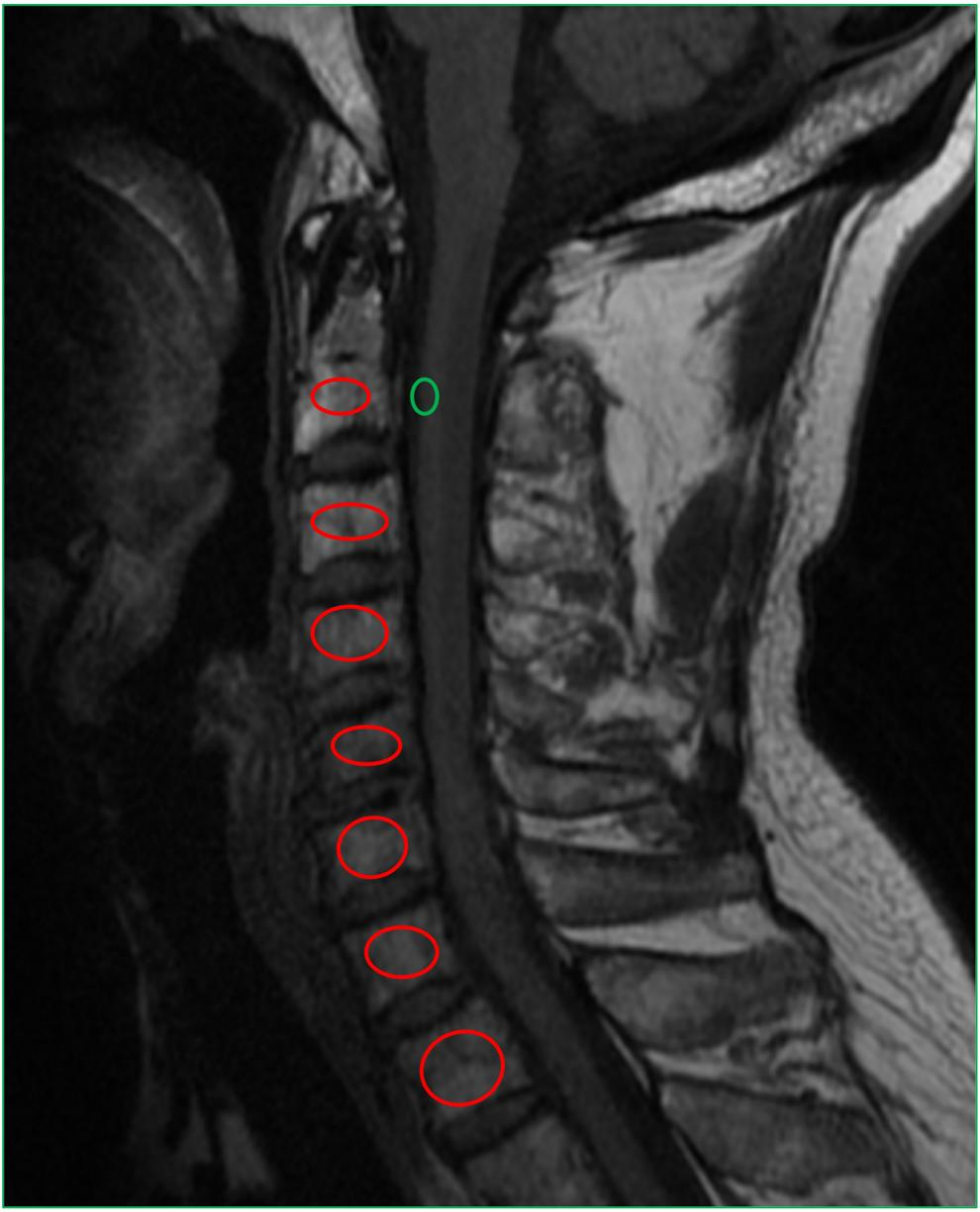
Sagittal T1-weighted magnetic resonance imaging (MRI) scan of the cervical spine (C2–T1) showing ROI placement for vertebral bone quality (VBQ) measurement. Red ROIs are placed within the central trabecular bone of each vertebral body (C2–T1), and an additional green ROI is drawn in the cerebrospinal fluid (CSF) region at the C2 vertebral level as the reference standard. The VBQ value is calculated as the ratio of vertebral body signal intensity to CSF signal intensity, providing a normalized index of bone marrow fat content and vertebral bone quality. Higher VBQ values indicate greater fatty infiltration and lower bone quality.
